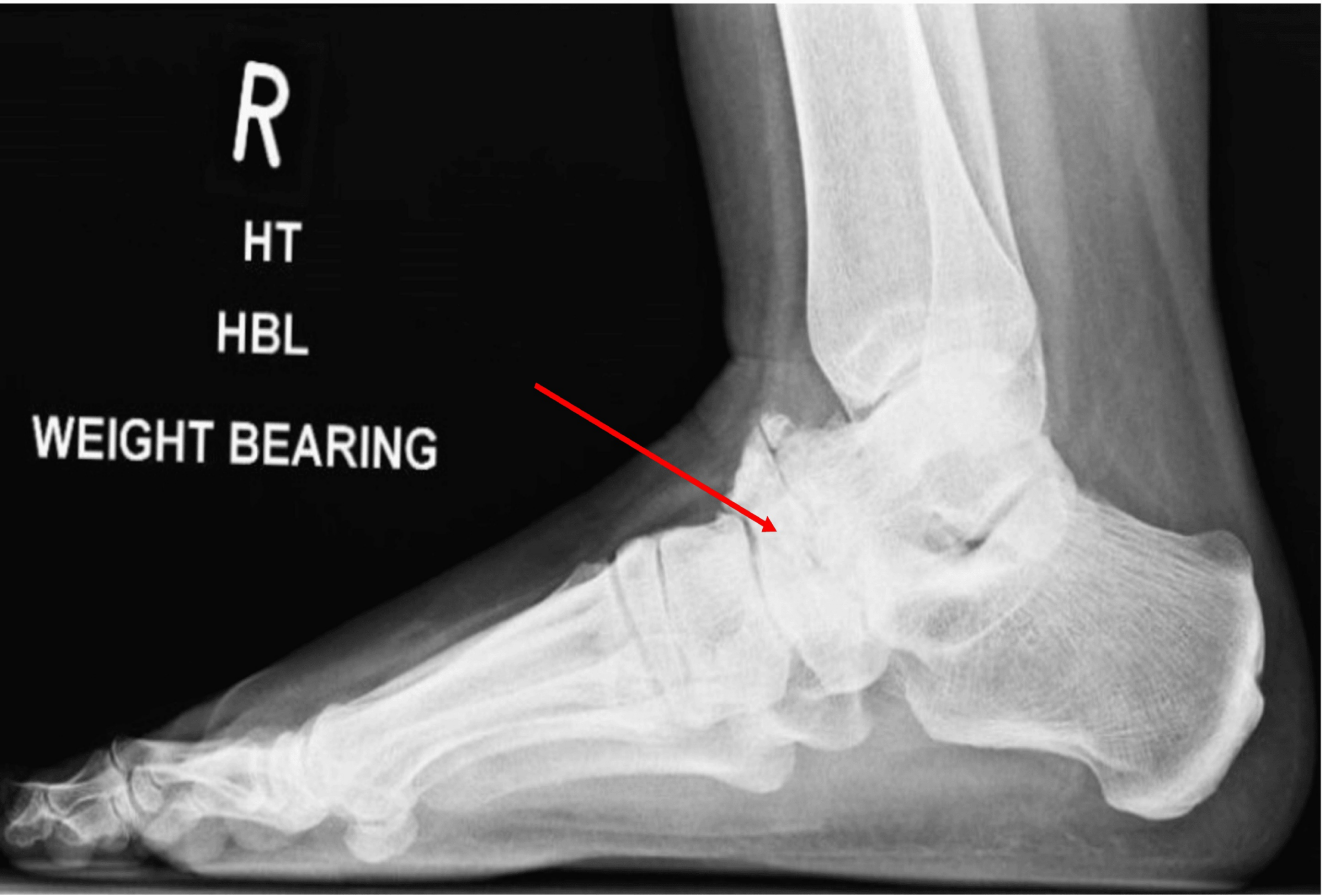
Lateral radiograph of the right foot, arrow demonstrates navicular collapse and arthritic changes
